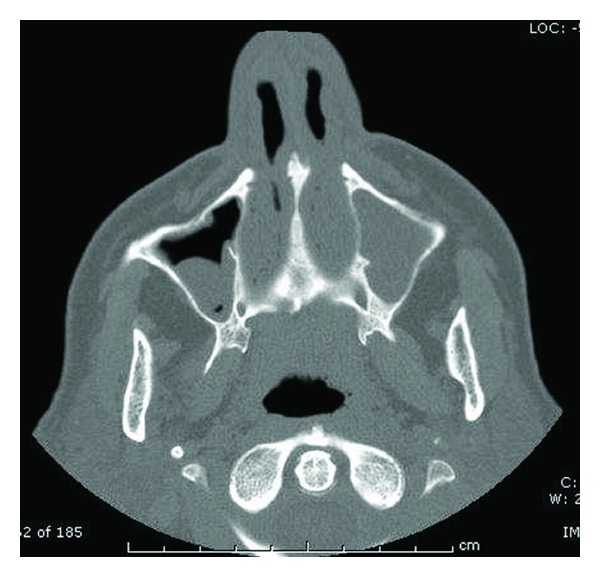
CT scan—mucous obliteration of the maxillary sinus (complete in the left, partial in the right); thickened and swollen nose soft tissues without osteostructural alterations.
